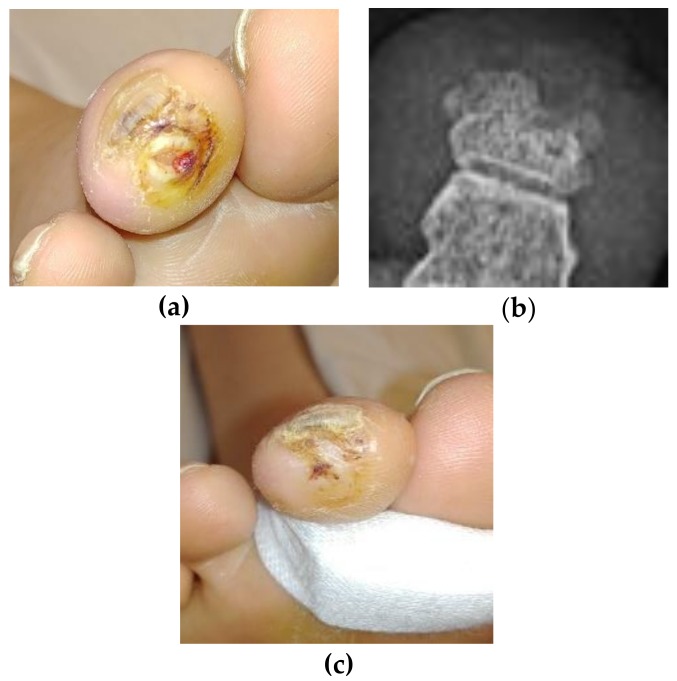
Progression of healing of the ulcer. (a) Image of ulcer at the distal tip taken on 30 April 2018. (b) Radiograph taken on 30 April 2018. (c) Image of closed ulcer at the distal tip taken on 8 May 2018.
